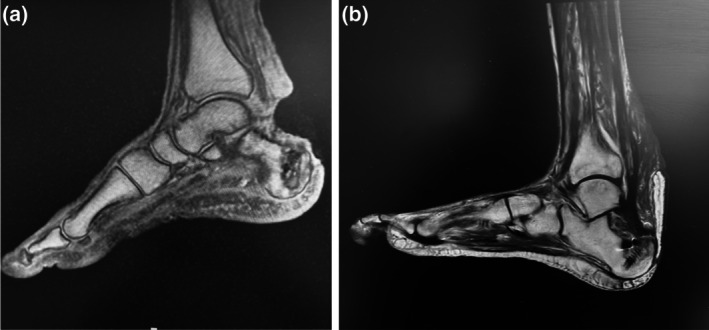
(a) Preoperative MRI showed extensive necrosis of the retro‐calcaneal area including most of the Achilles tendon, proximal displacement of the Achilles tendon proximal stump, severe erosion of the calcaneal bone, and possible FHL tendon involvement; (b) 6 months postoperatively MRI shows complete restoration of the Achilles tendon.
